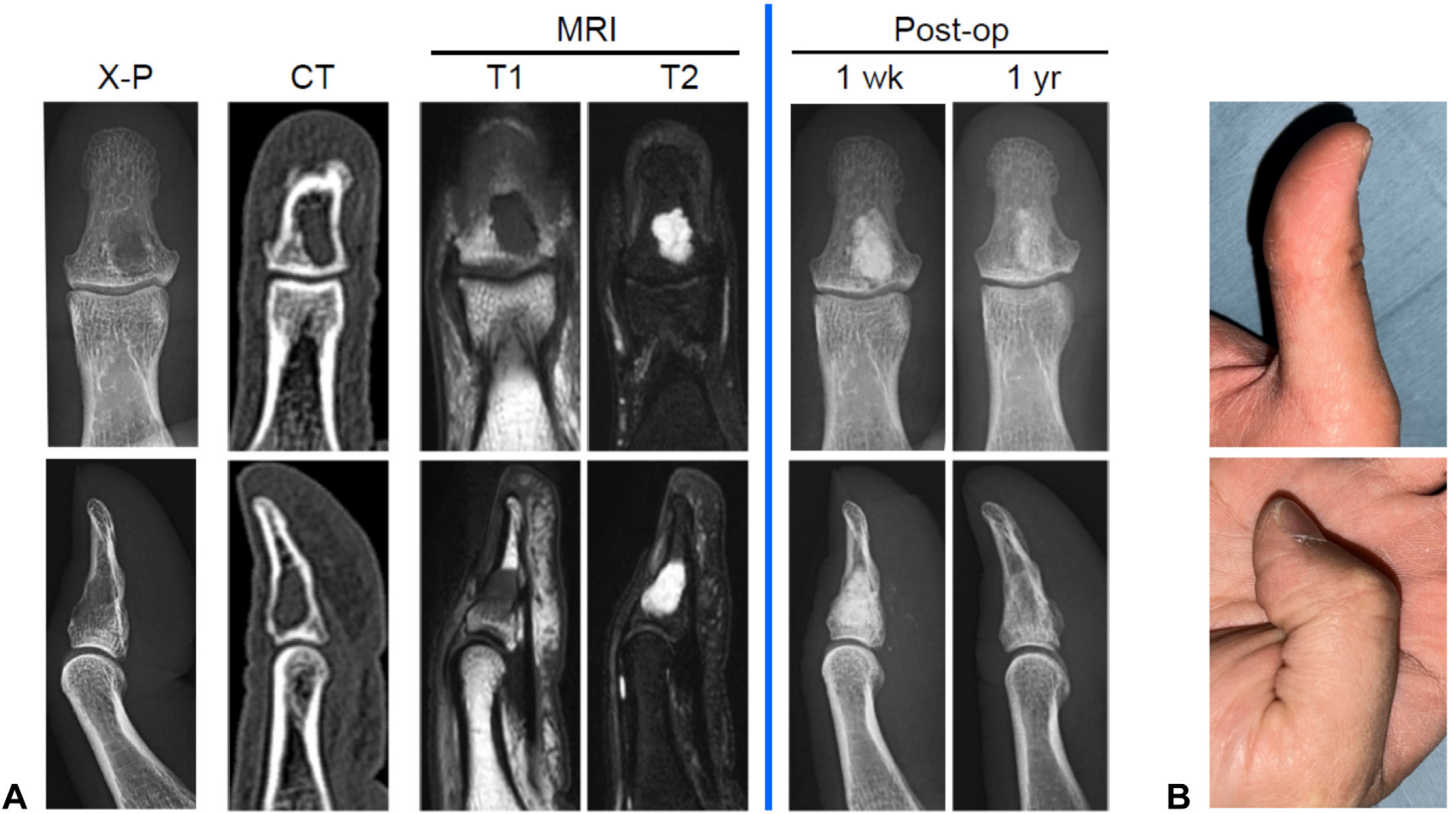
Pre- and postoperative findings for case 1. A Left of the blue line: Preoperative radiographs, computed tomography, and magnetic resonance imaging (T1- and T2-weighted images), showing frontal (upper row), and sagittal (lower row) views. Right of the blue line: Postoperative radiographs at 1 week and 1 year, with frontal (upper row), and lateral (lower row) views. B Thumb range of motion at 1 year after surgery: extension (upper) and flexion (lower).
